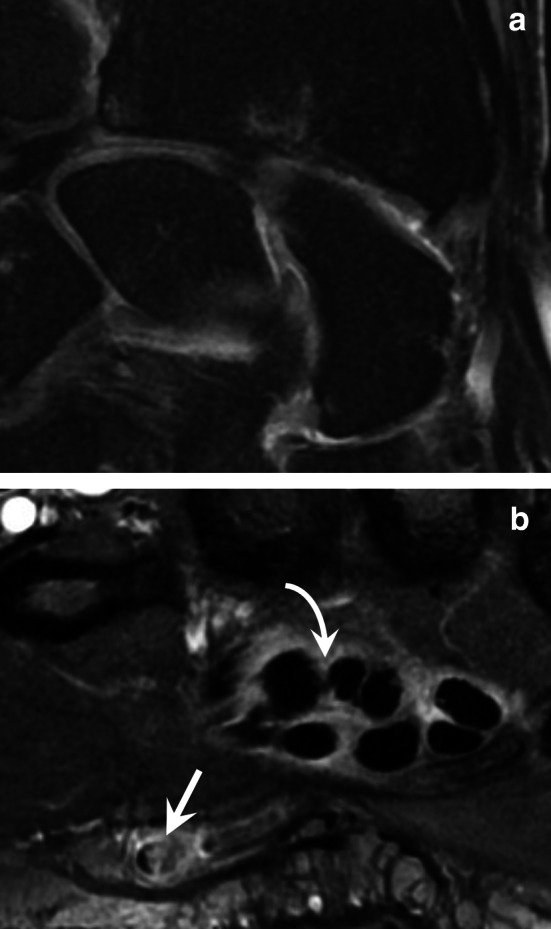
HAVS in a 62-year-old man. a Coronal T2-weighted MR image showing isolated osteoarthritis of the radioscaphoid compartment of the radiocarpal joint. b Contrast-enhanced T1-weighted fat-suppressed sequence showing a focal occlusion at the junction of the UA and the SPA (straight arrow) and a tenosynovitis of the flexor digitorum tendons (curved arrow). The UA was otherwise preserved
